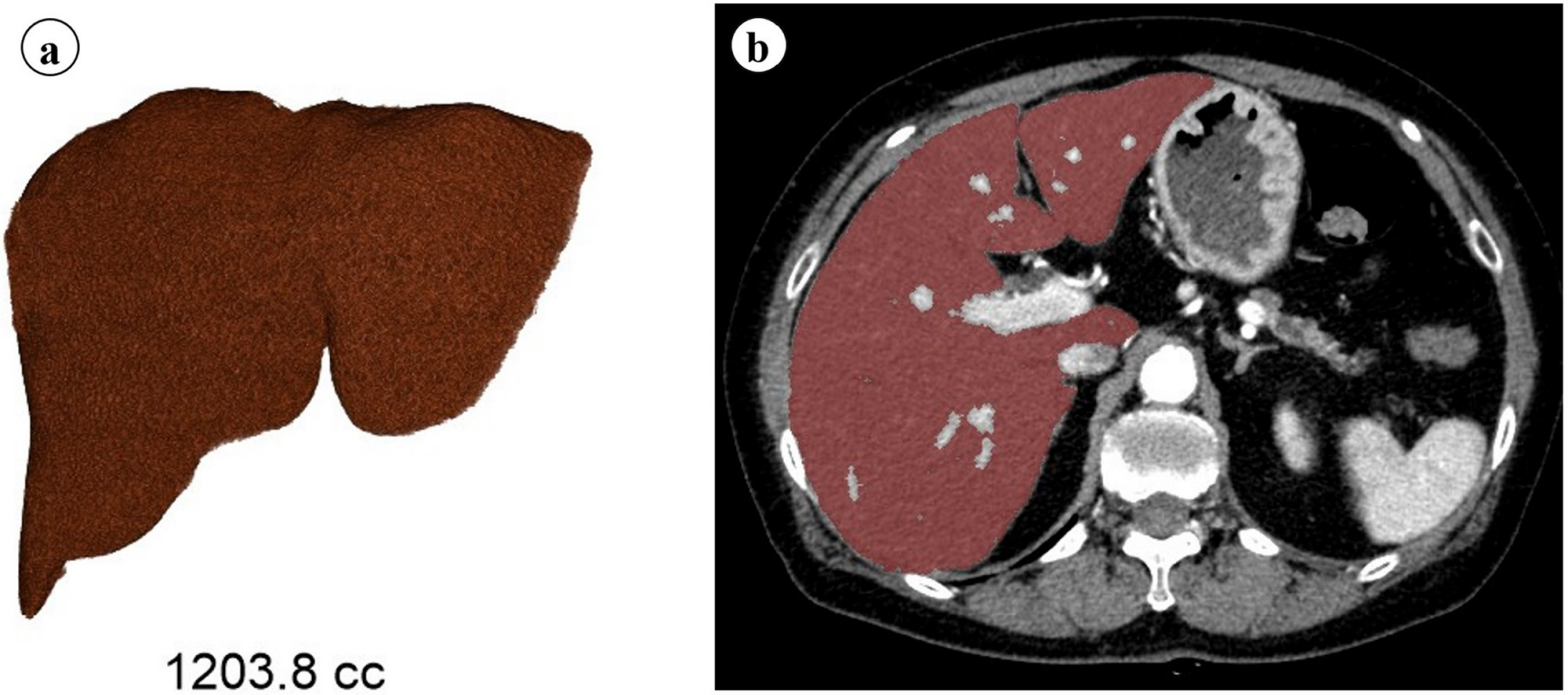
CT volumetry of the liver in the portal venous phase. The image on the left shows volumetry whereas the image on the right shows a mask image.
